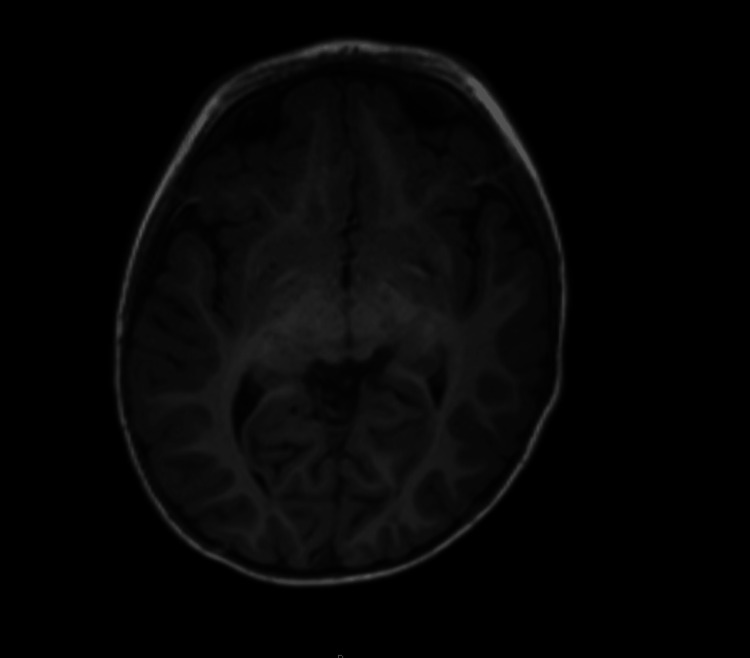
Axial T1-weighted magnetic resonance imaging (MRI) of the brain demonstrating no acute infarction, mass effect, hemorrhage, or structural abnormalities. The temporal lobes are unremarkable.
